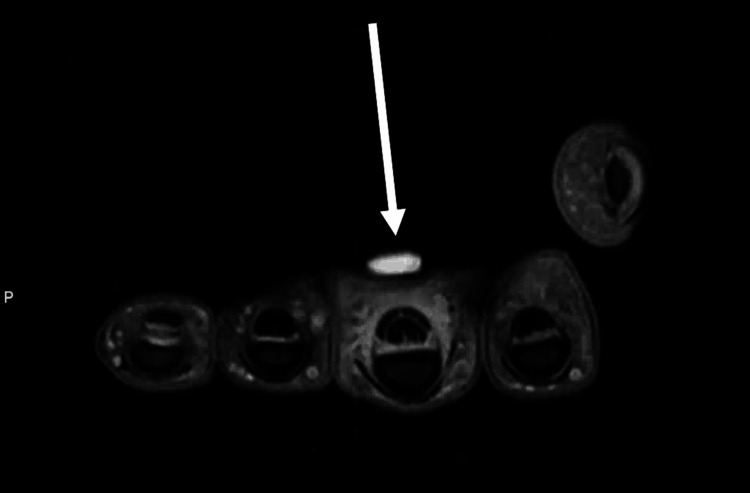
Preoperative MRI axial image of the left hand There was noticeable swelling in the left long finger flexor tendon sheath.
